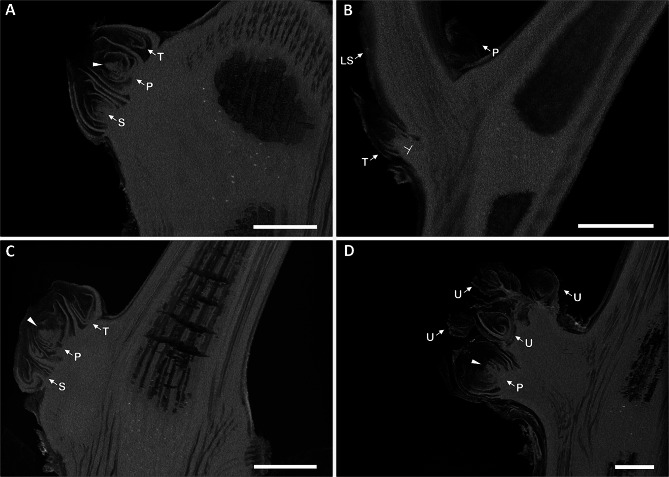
Representative CT scans of buds from (A) Dakapo WT, (B) Dakapo WB, (C) Merlot WT, and (D) Merlot WB samples. Primary (P), secondary (S), and tertiary (T) bud primordia are indicated if present in the image. The inflorescence primordia are indicated by the solid triangle in the (A) Dakapo WT, (C) Merlot WT, and (D) Merlot WB samples. Only one inflorescence primordium is present in the images, although multiple were seen for both WT samples. The Merlot WB sample shown (D) is the only Merlot WB sample scanned with a potential inflorescence primordium present, although the inflorescence primordium seen appears to be deformed due to the edges being smoother than those seen in WT samples (A and C). The lateral shoot stem (LS) is indicated in (B) Dakapo WB. The bud primordia in the Merlot WB sample shown are challenging to accurately label, aside from the more-developed primary primordia (P), so we have labeled the additional bud primordia as uncharacterized primordia (U) in (D) Merlot WB, which are indicated as well. Scale bar = 1 mm
